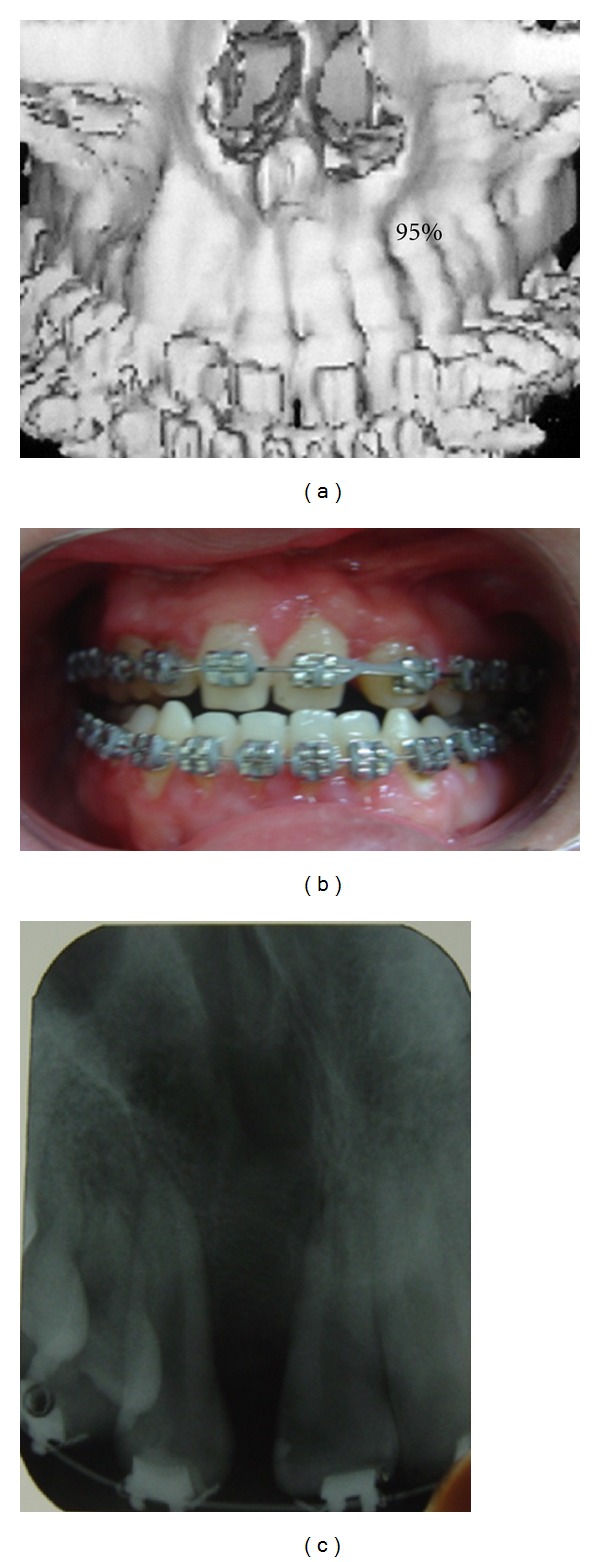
Patient with grade I. (a) CT scan view; (b) occlusal view; (c) dental X-ray.
